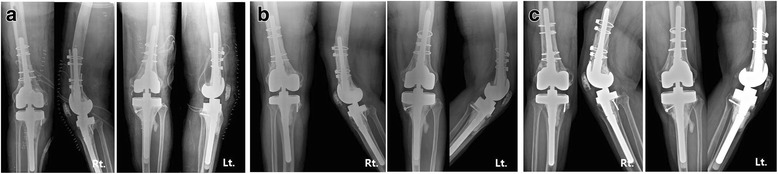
A series of radiographs. a anteroposterior and lateral radiographys of both knees at post RTKA, b 5 years follow-up, c 10 years follow-up showing good allograft incorporation
